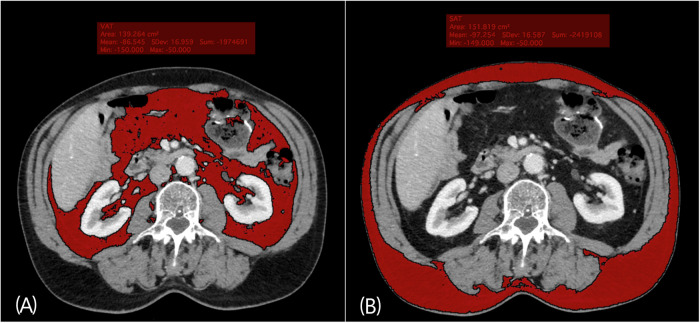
Determination of abdominal adipose tissue area by quantitative CT-scan. The analysis was performed at L3-level at the point where both transversal processes were visible. Adipose tissue areas were indicated by Hounsfield scale between −150 and −50 HU, which is an attenuation value specific for fatty tissues. Red color marks (A) VAT-area (B) SAT-area. Direction of scanning view: Top-down.
